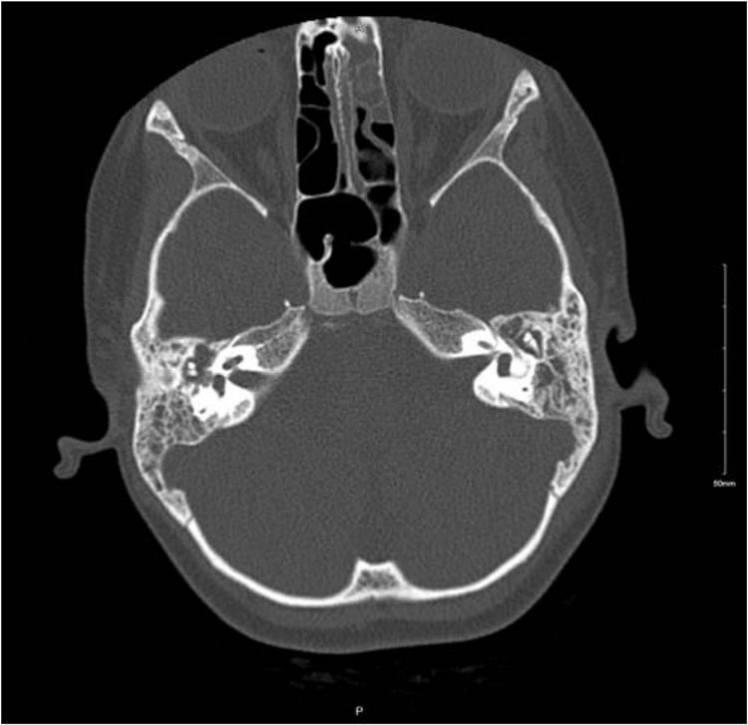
Non-enhanced axial CT scan of temporal bone showing bony remodeling suggestive of chronic otomastoiditis.
